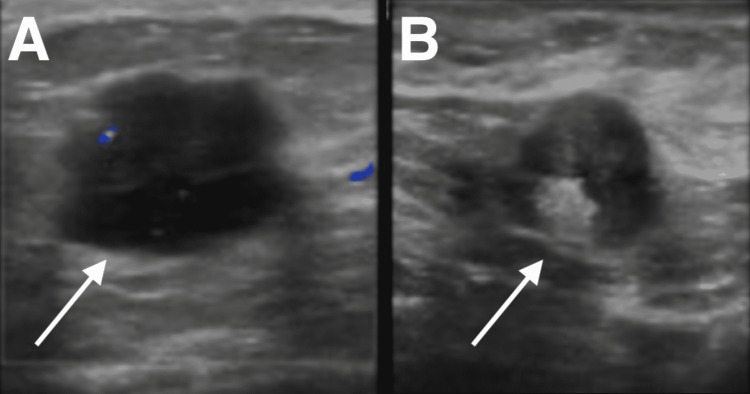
Ultrasound of the breast showing (a) irregularly-shaped, hypodense, retroareolar mass (arrow), (b) enlarged ipsilateral axillary lymph node with lobulated contour, increased width, widest diameter of 2.8 cm, with preserved hilum (arrow)
